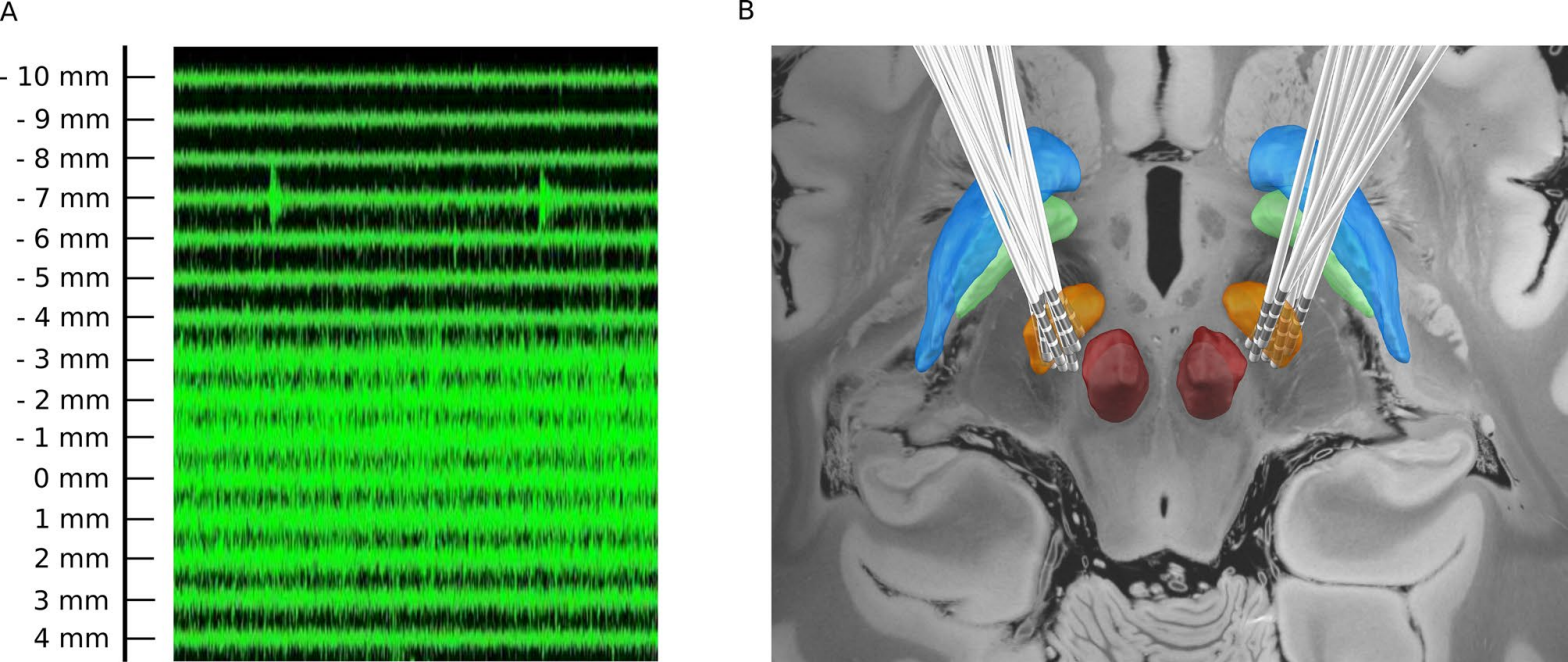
(A) Raw microelectrode recordings (MER) obtained from different depth above and below the target. (B) Final electrode positions in all patients, using Lead-DBS 2.0 (https://www.lead-dbs.org/). Subcortical structures are based on DISTAL atlas (Orange, STN; Green, GPi; Blue, GPe and red, red nucleus) and the background template is 7 T MRI ex vivo 100 micron human brain.
